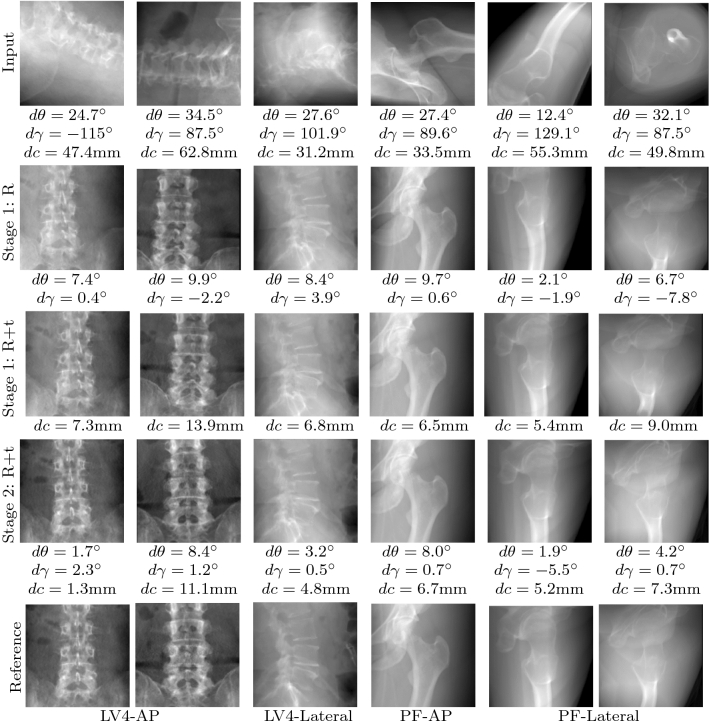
Qualitative evaluation of the sequential pose prediction on synthetic X-rays. For comparison, the last row shows the reference standard defined by the expert
